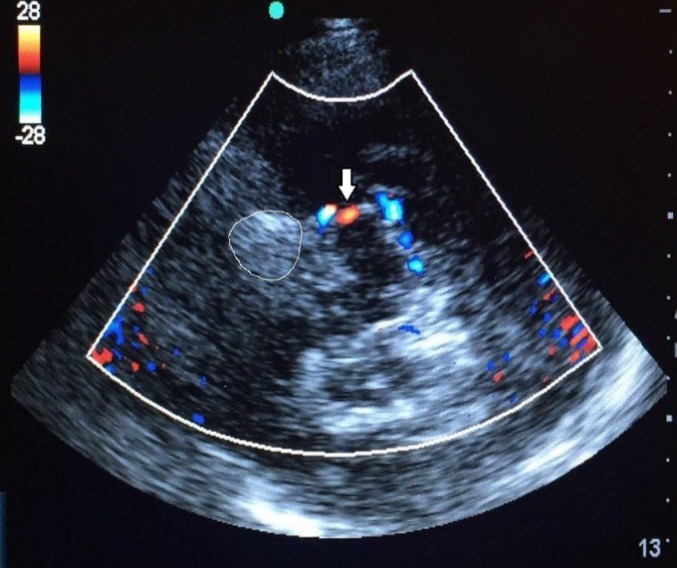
Axial transcranial color sonography (mesencephalic level); coiled aneurysm was encircled. There was no refilling within the aneurysm. Arrow indicates posterior cerebral artery (PCA).
